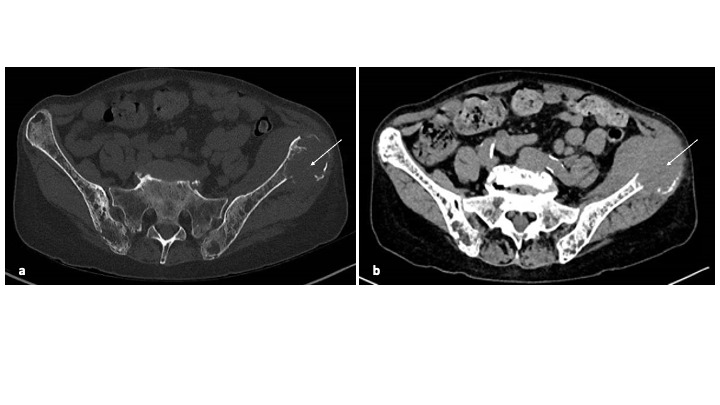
Pelvic CT-scan after two weeks of treatment, in bone window (a) and soft tissue window (b), showing a large lytic lesion of the left iliac crest invading soft tissues (white arrow)
